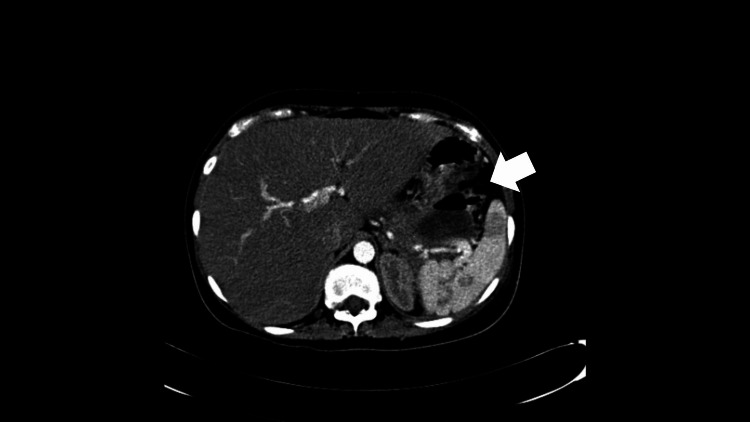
Triphase CT scan of the abdomen - axial view, arterial phase The image shows an organized heterogeneous left retroperitoneal collection with multiple loculations extending to the left pelvis with air specs tracking the skin over the left anterior superior iliac spine; a few air specks are also seen in the pancreatic tail CT: computed tomography
